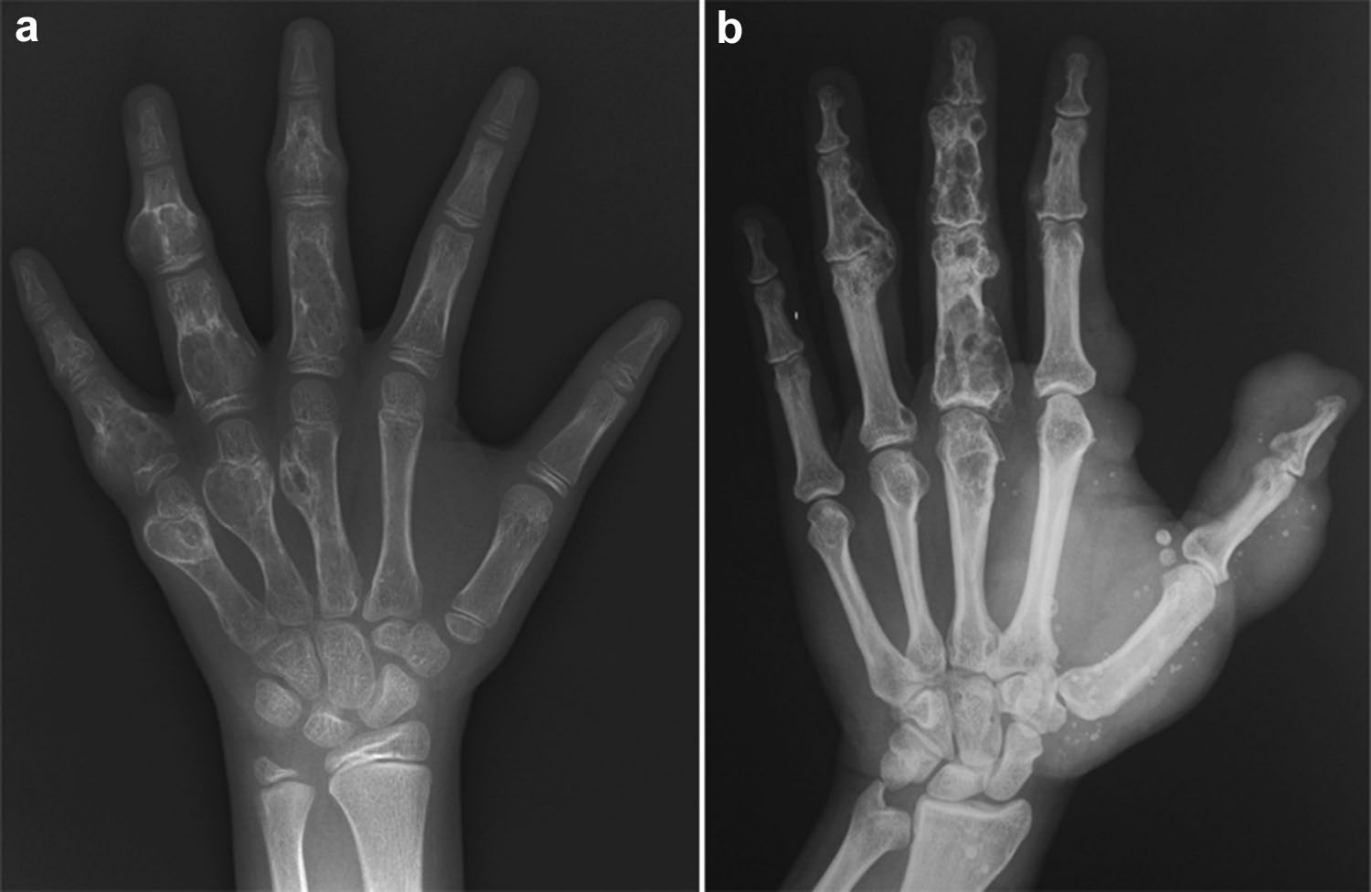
a An 8-year-old child with enchondromatosis (Ollier disease). Radiograph shows multiple expansile metaphyseal lucent lesions in the third to fifth phalanges. b A young adult with Maffucci syndrome. Radiograph shows multiple enchondromatosis in the second to fourth digits with soft tissue masses associated with multiple foci of round phleboliths compatible with hemangiomas
